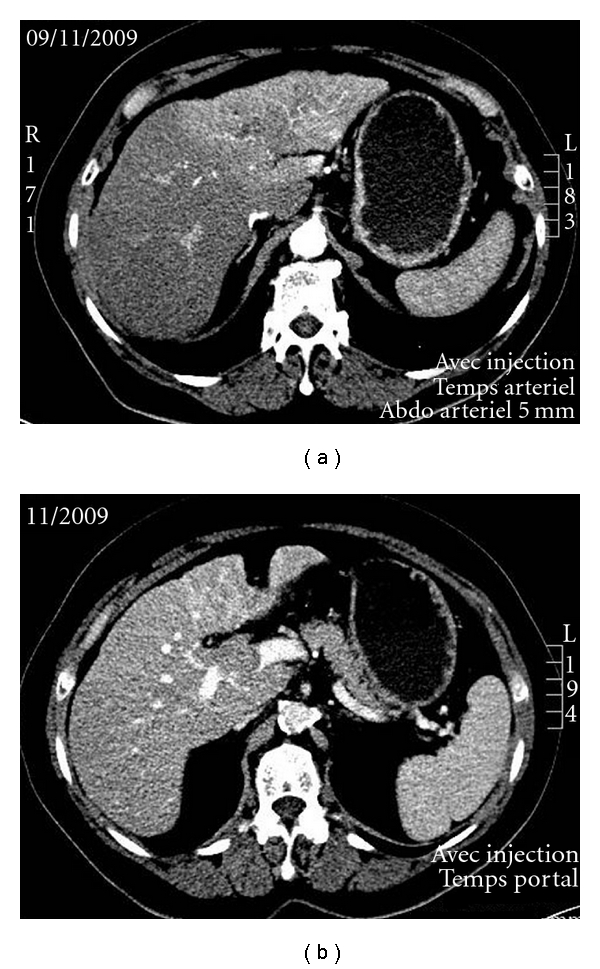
case report: patient with a large HCC Contrast enhanced CT evidencing a large infiltrative tumor of the left liver (a) with a portal vein thrombosis (b).
